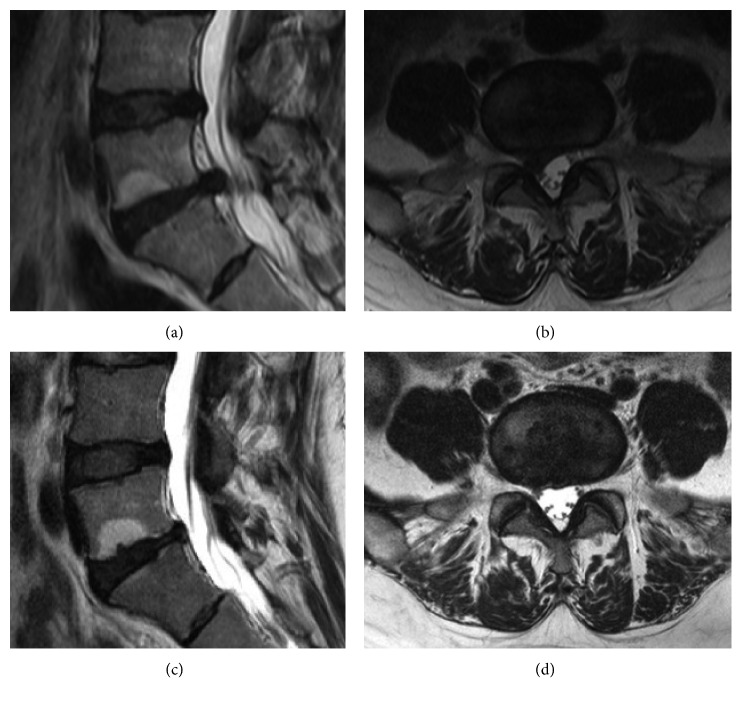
Case presentation regarding change of protruded disc volume after the SELD procedure. The 38-year-old man suffered from right leg pain with the protruded disc at L5/S1 level. After the SELD procedure, the volume of the protruded disc at the L5/S1 level decreased. (a) Preoperative sagittal T2 MR image; (b) preoperative axial T2 MR image; (c) postoperative sagittal T2 MR image; (d) postoperative axial T2 image. SELD = trans-sacral epiduroscopic laser decompression; MR = magnetic resonance.
